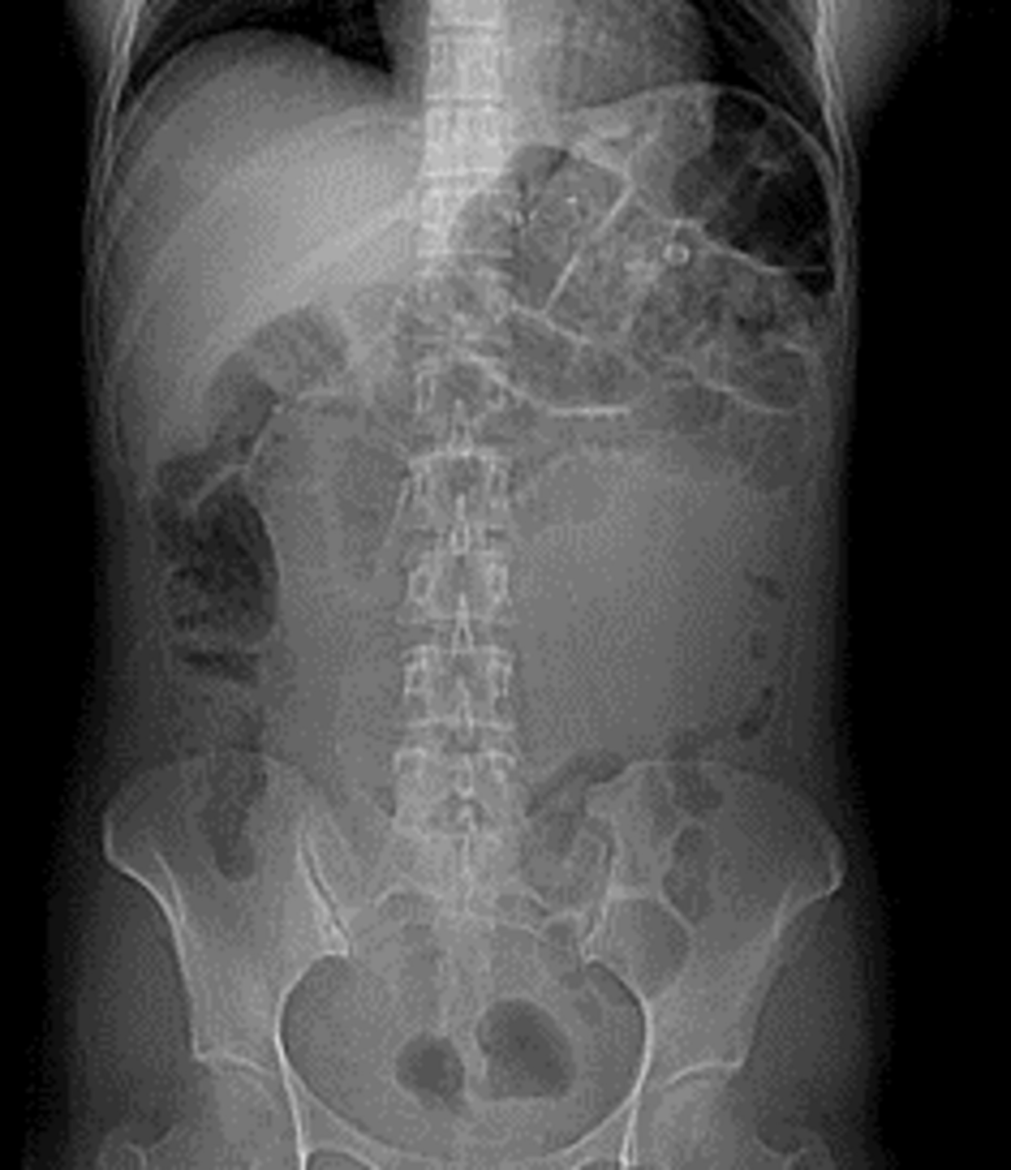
Upright X-ray abdomen showed dilated bowel loops in the left upper quadrant
